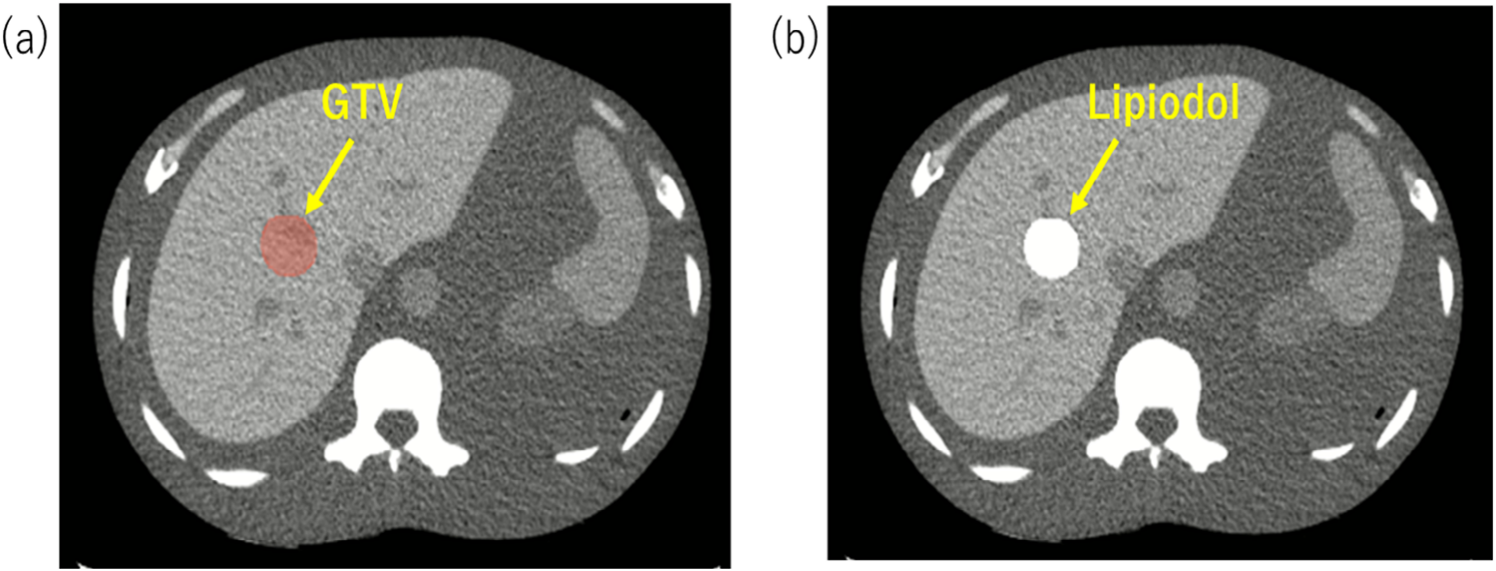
(a) Virtual tumor region of a 3 cm sphere defined as the GTV on the CT image. (b) Lipiodol region assigned to 10 000 HU in the GTV.
